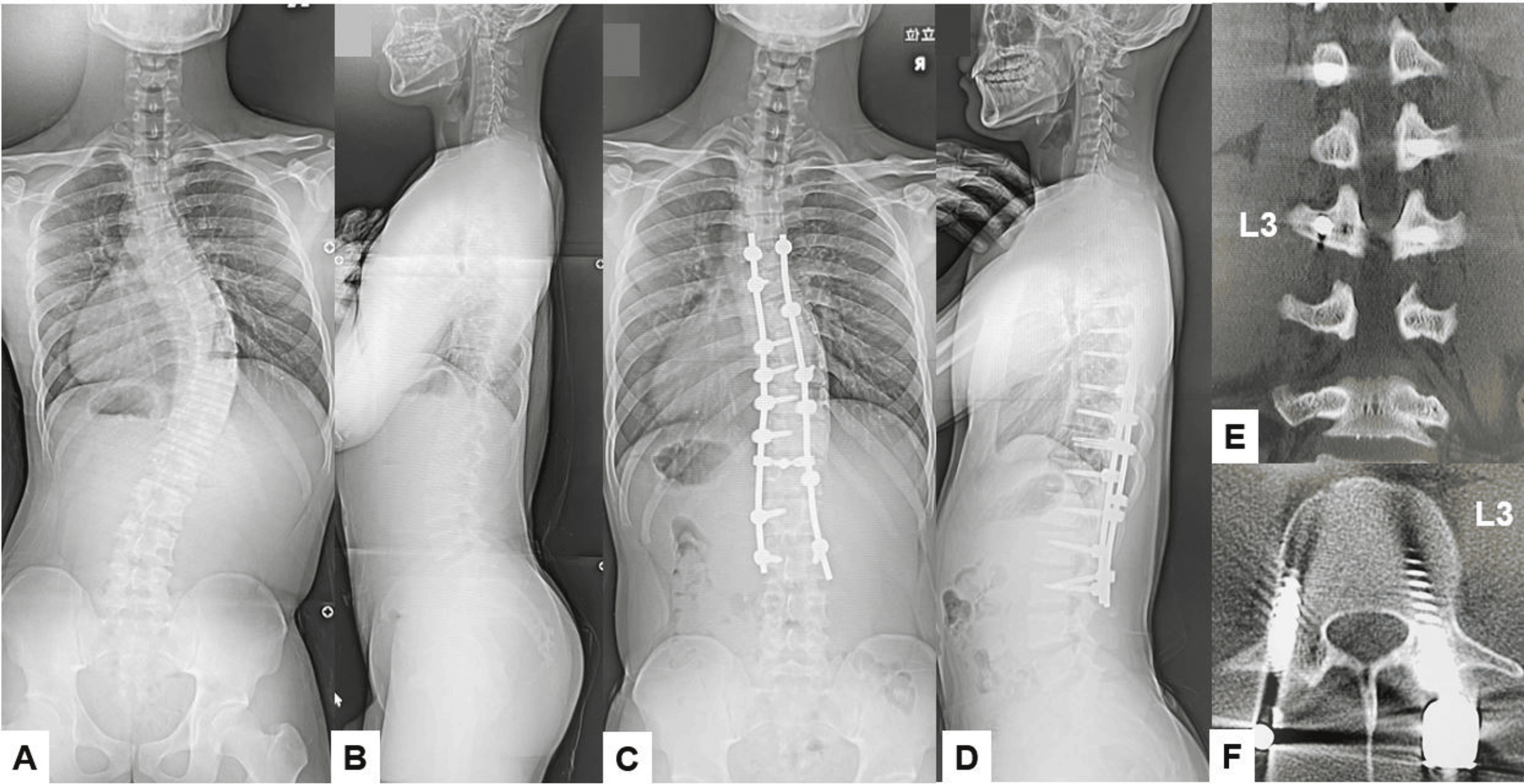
Pre-and postoperative images A: Preoperative posteroanterior whole spine radiogram, B: Preoperative lateral whole spine radiogram, C: Postoperative posteroanterior whole spine radiogram, D: Postoperative lateral whole spine radiogram, E: Coronal reconstruction lumbar CT, and F: Axial lumbar CT at L3.  The Cobb angle was corrected from preoperative 56 degrees to postoperative 23 degrees.
